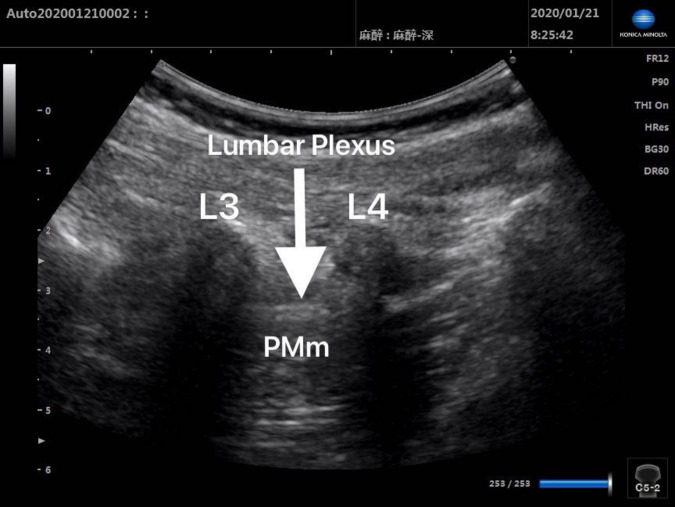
Postinjection ultrasound image of L3-4 lumbar plexus block.
